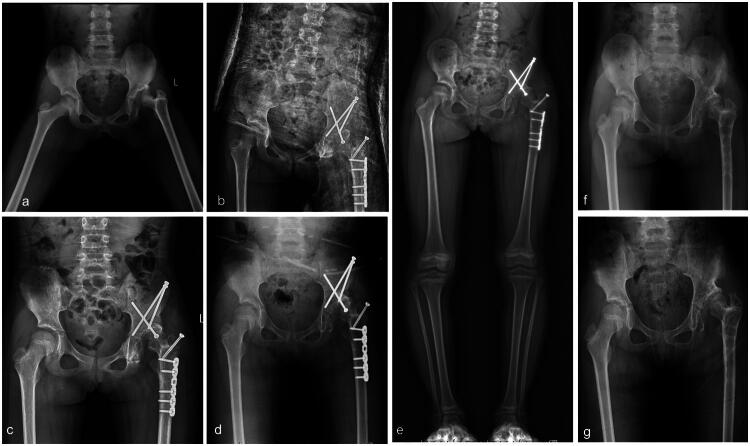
a–g The case 4, a child aged 7–8 years, developed left-sided SAH at 3–4 years of age. The inflammation was effectively controlled with antibiotics, hip arthroplasty, and drainage at other institutions. One year ago, she developed claudication and pain in the left hip. A pelvic X-ray showed a subluxation of the left hip with partial resorption of the femoral head (type IIA) (a). She underwent a pemberton osteotomy of the acetabular side combined with GTE and a proximal femoral osteotomy (b). Postoperatively, the osteotomy site healed, the hip joint was stable, the acetabulum was satisfactorily accommodated, there were no residual complications such as LLD, and the hip joint functioned well more than one year after surgery (c–e)—removal of internal fixation devices (f and g).
